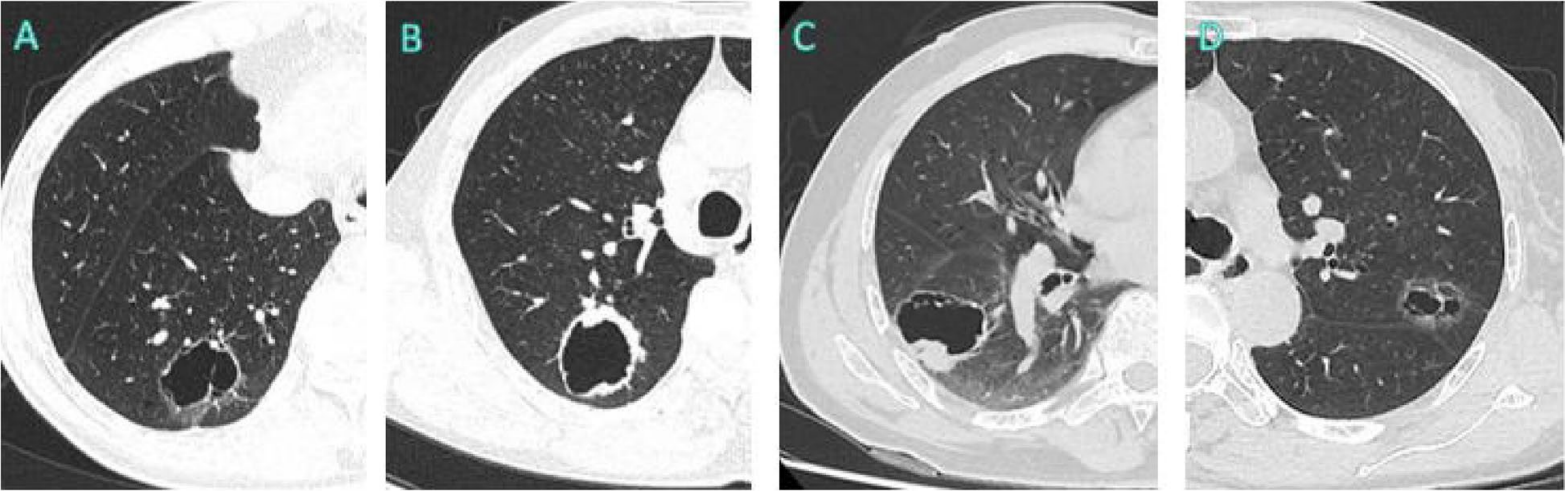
CT images of four morphological patterns of cystic pulmonary nodules. A Type I, mean thin wall(< 2 mm); B type II, mean thick wall(≥ 2 mm); C type III: a cystic airspace with a mural nodule; D type IV, tissue intermixed within clusters of cystic airspaces
